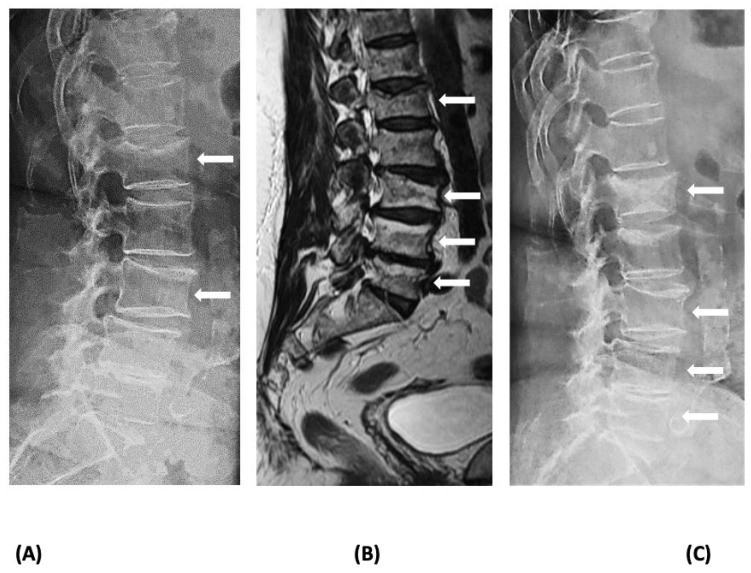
Imaging of a patient with rebound-associated vertebral fractures 10 months after the last denosumab dose (4 months off treatment): (A) lateral lumbar spine X-ray at the time of acute back pain onset showing fractures at L1 and L3; (B) magnetic resonance imaging of the spine at the same time reveals additional recent fractures at L4 and L5; and (C) lateral lumbar spine X-ray 3 months later depicting deterioration of the deformities of the vertebrae (collapse of the acute, non-collapsed vertebral fractures). Fractures are indicated with white arrows.
